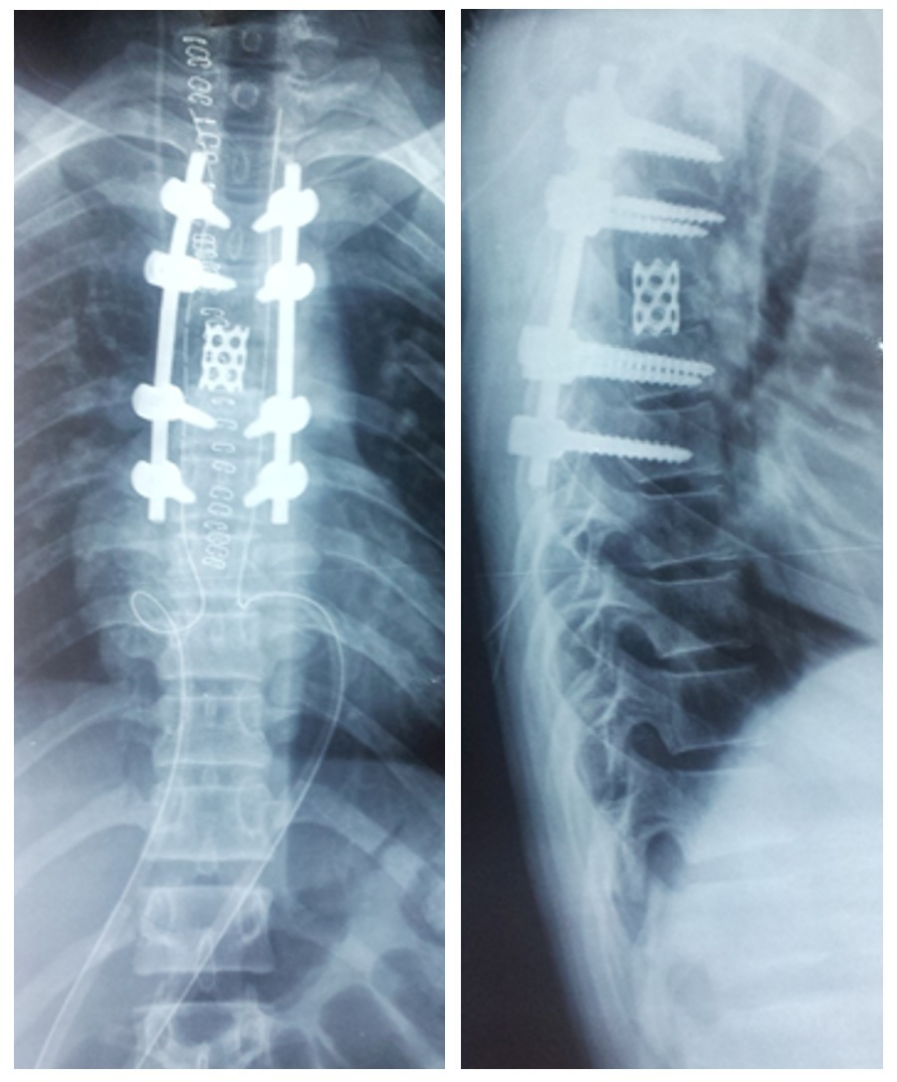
Intraoperative anteroposterior and lateral view x-ray of the thoracic spine showing cage and pedicle screw fixation
